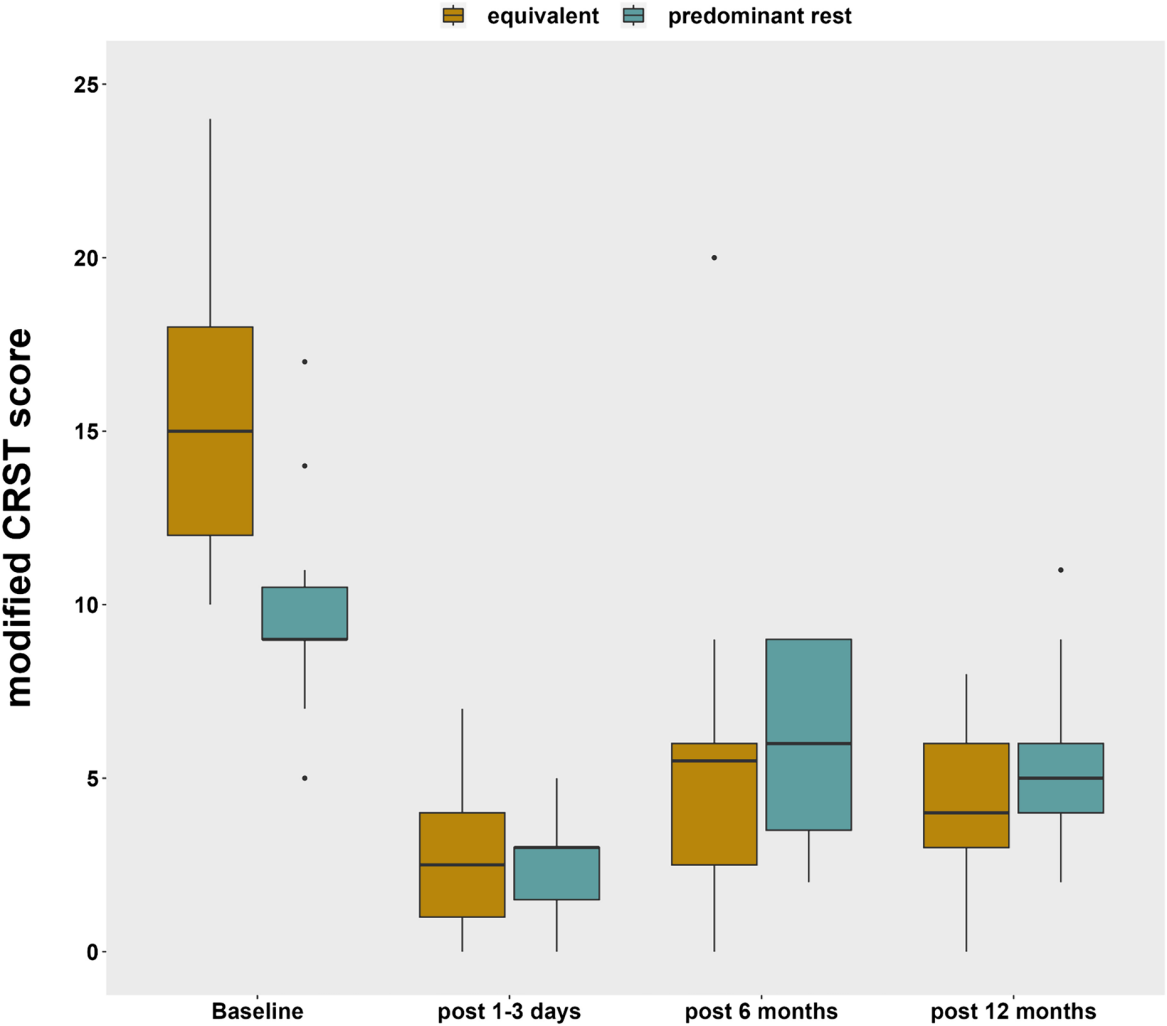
Tremor improvement after MRI-guided focused ultrasound (MRgFUS) thalamotomy in patients with concomitant rest and postural and predominant rest tremor subtype. 12 months post-MRgFUS, significant tremor improvement was observed in the treated extremity—as shown by modified, hand-specific subscores of the Clinical Rating Scale of Tremor (CRST)—in patients with concomitant rest and postural tremor only
